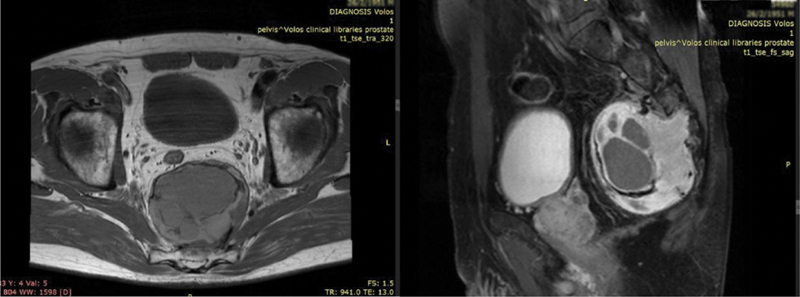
Lower abdomen magnetic resonance imaging (MRI). A well-circumscribed heterogeneous signal intensity mass arising from the intraforaminal segment of the S3 nerve root, measuring 7.5 × 6.4 × 7.7 cm.
